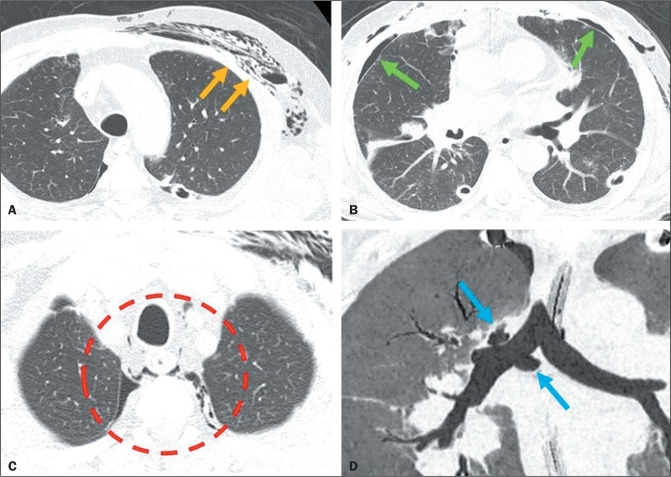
Signs of post-LT air leakage on CT. A: Emphysema in the left chest wall
